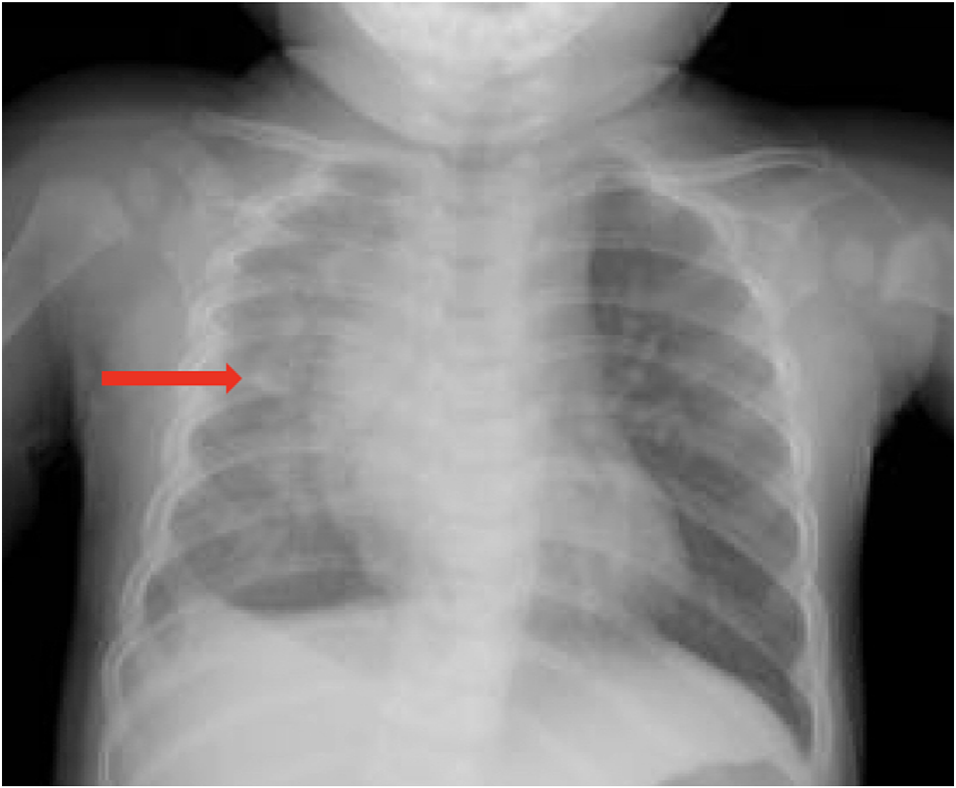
Chest radiograph on day 3, following return to the emergency department. Right lung consolidation suggestive of pneumonia is visible.
